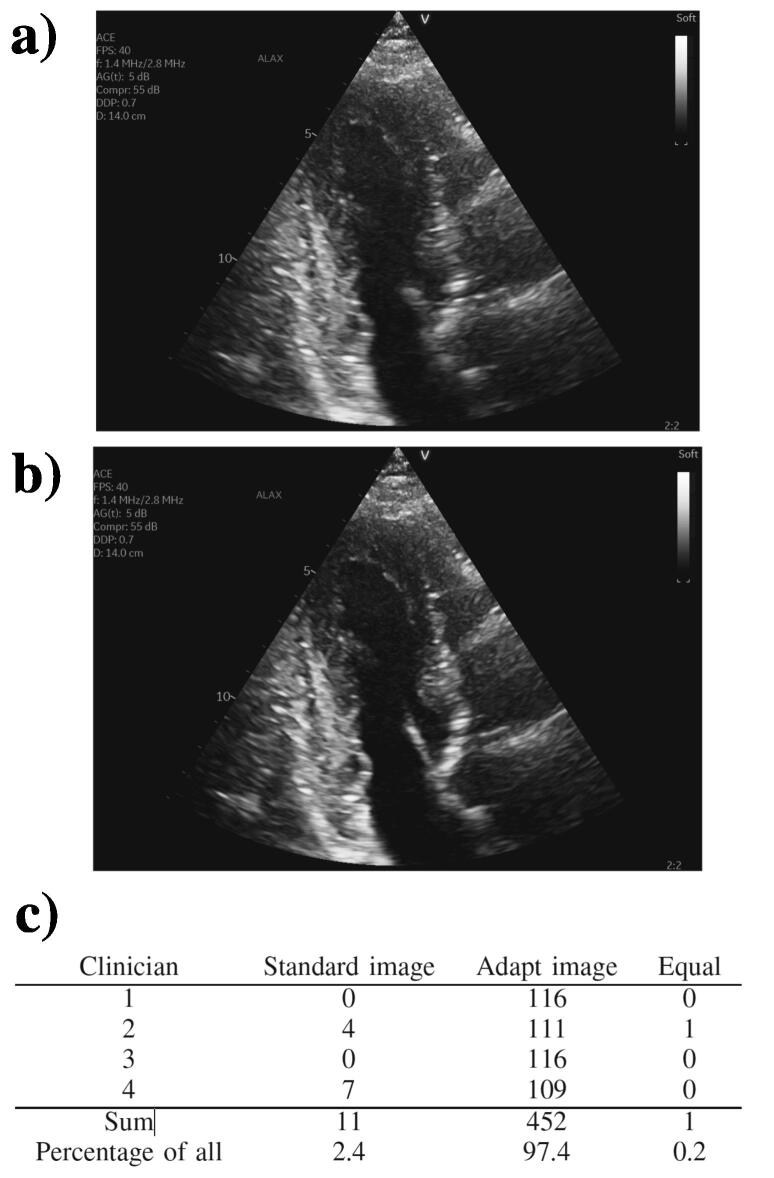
Aberration correction in 2D echocardiography (Måsøy et al. 2022 [170]). a) and b) shows apical long axis (ALAX) views from a patient with standard imaging (a) and after aberration correction (b). After aberration correction, the cardiac structures, including endocardiac borders and valves, appear thinner and more clearly defined. In the example, the mitral valve leaflets and endocardial borders become very clear after aberration correction. c) Shows a side-by-side clinical comparison of standard imaging and aberration corrected images (Adapt). The two last rows summarize the results as the accumulated sum for each column, and the percentage of each category with respect to all evaluated cineloops. Reproduced from [170] (Fig.5, Table 1) under License CC BY 4.0.
